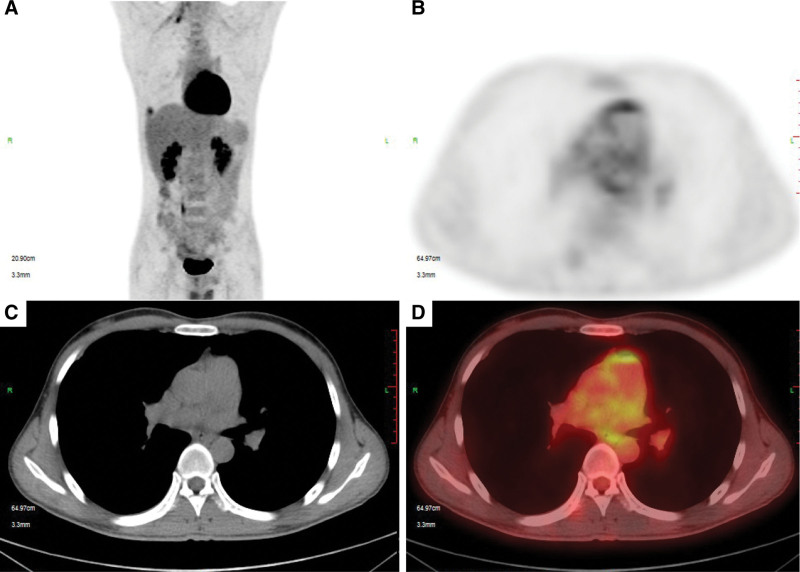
PET-CT imaging results of a patient with TA. PET-CT = positron emission tomography-computed tomography, TA = Takayasu arteritis.
